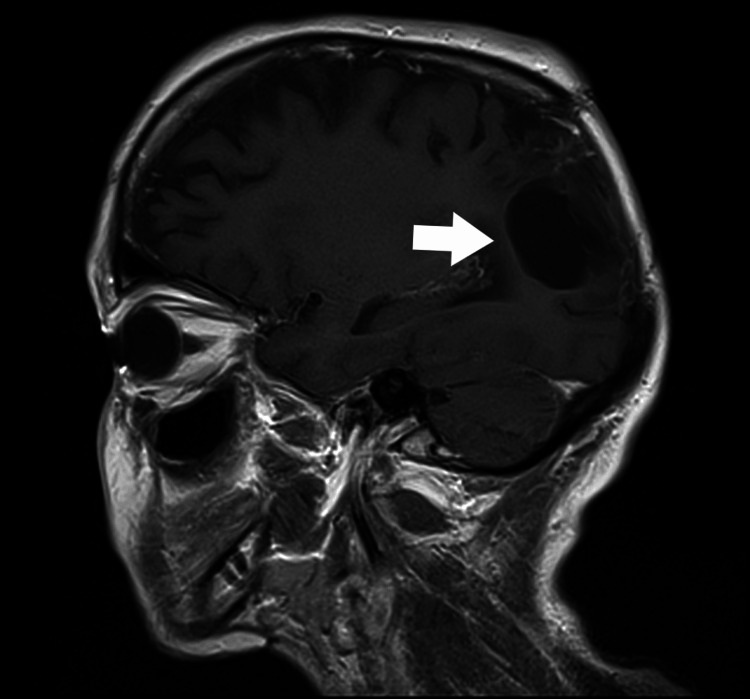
A follow-up MRI scan of the head 1.5 years after surgery: a side view. No signs of recurrence of the abscess (marked with a white arrow).
